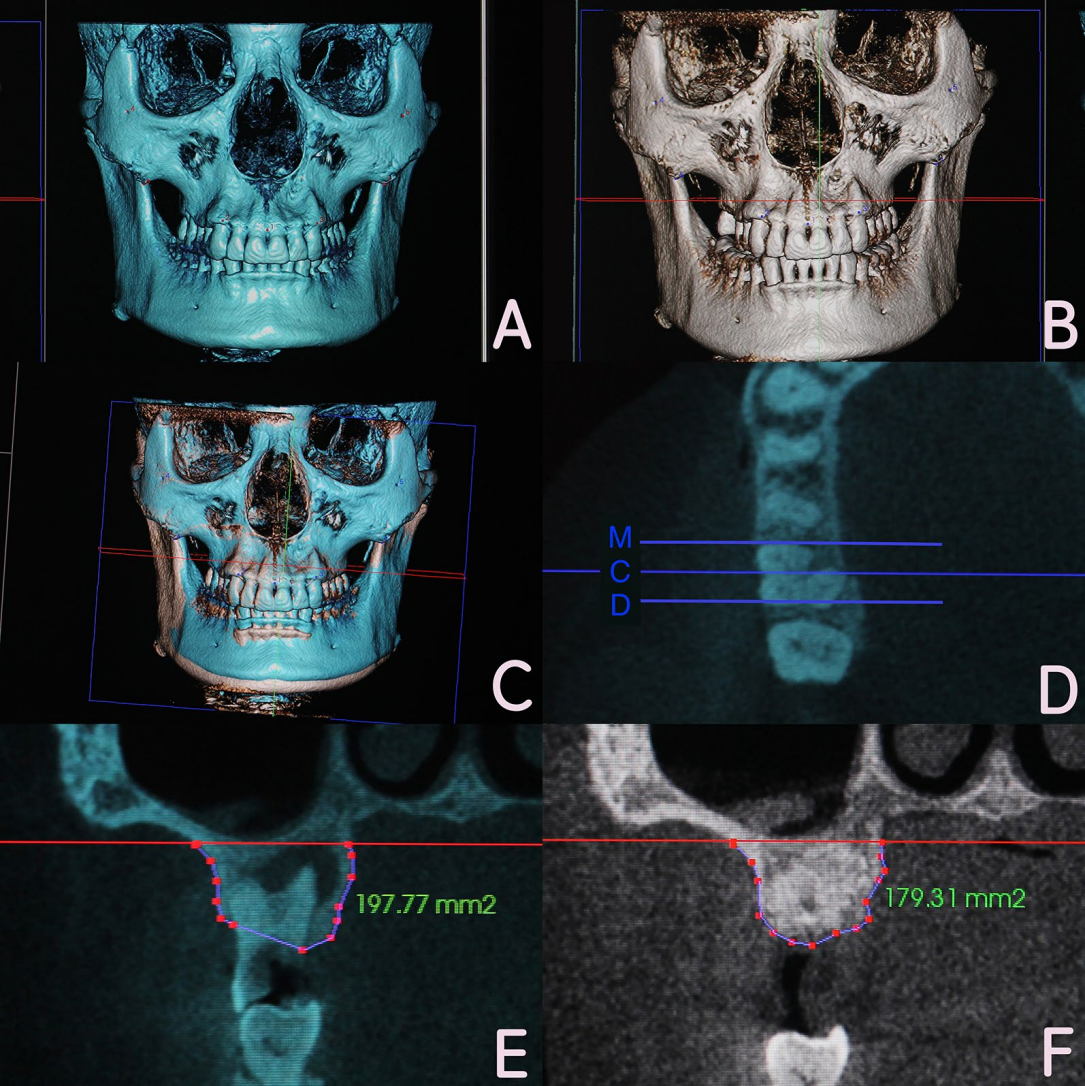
CBCT observation and measurement method. a Preoperative three-dimensional image; b 8-month postoperative three-dimensional image; c three-dimensional image after superimposition; d C-, M-, D-section were determined on the superimposed images; e the preoperative alveolar bone area was measured; f the 8-month postoperative alveolar bone area was measured
